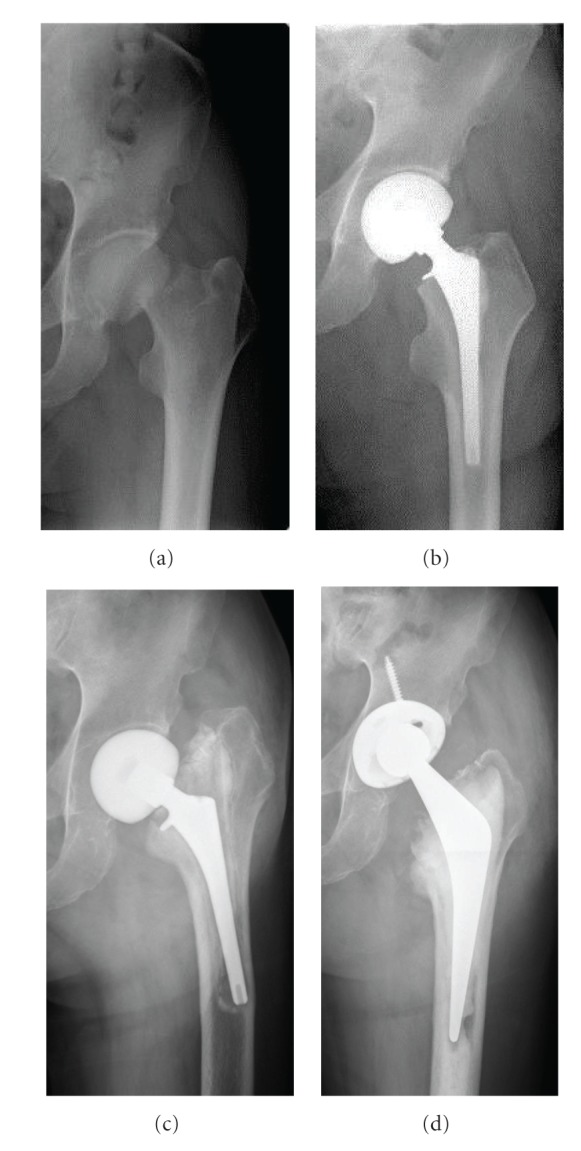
Radiographical findings, (a) before operation of femoral head replacement, (b) after operation of femoral head replacement, (c) 1 week after operation of femoral head replacement.
